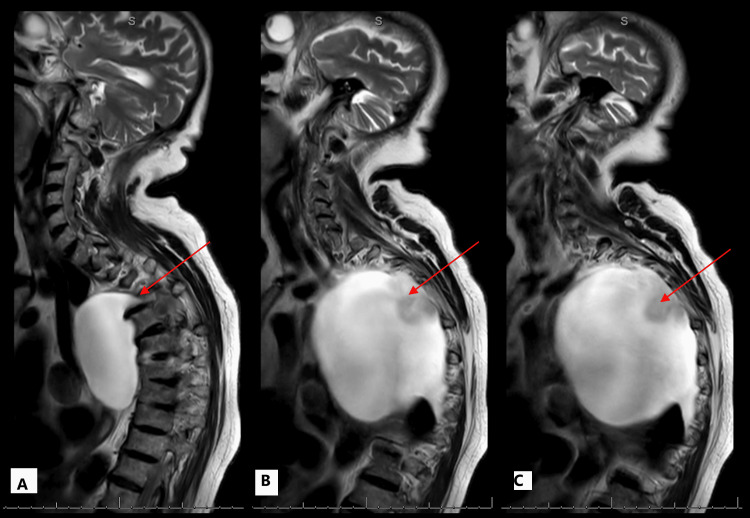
Sagittal T2 weighted MRI images
